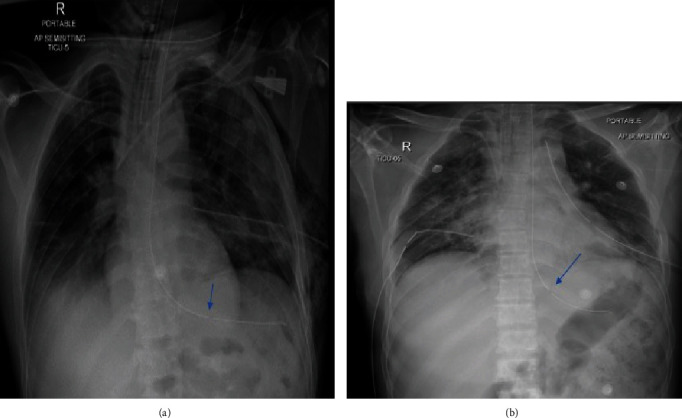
Chest X-ray (a & b) shows endotracheal tube, orogastric tube and left thoracostomy tube: The side hole of the feeding tube seen overlying the gastro-esophageal junction (arrows).
